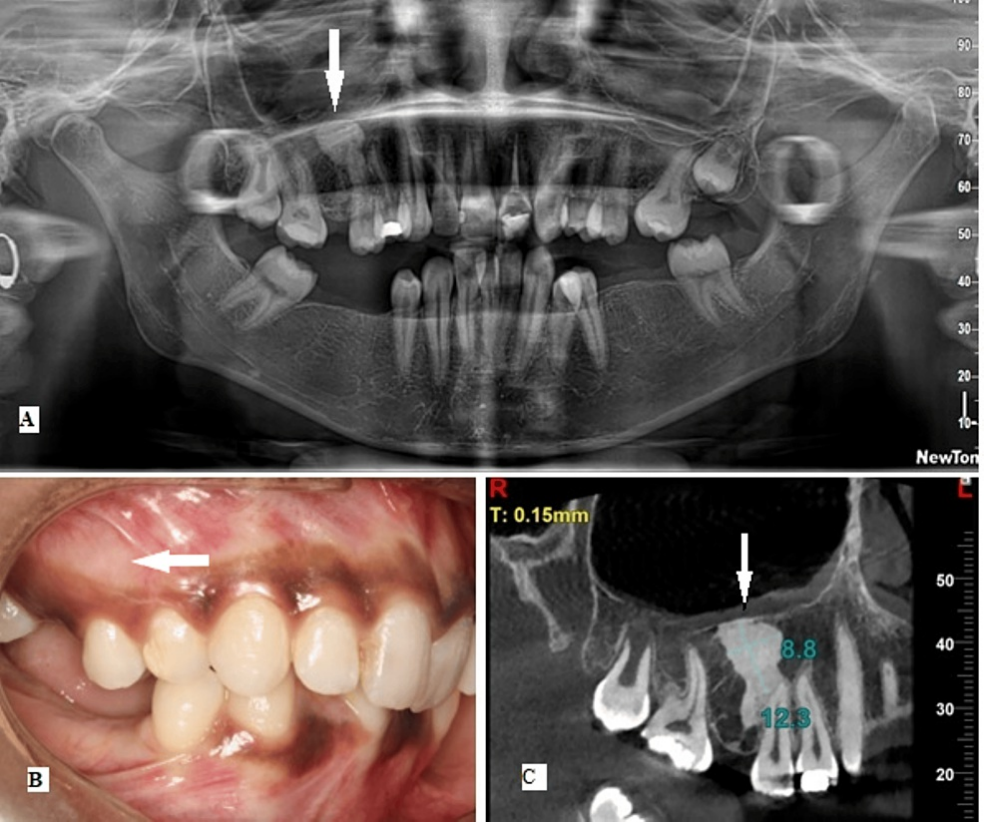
A 19-year-old female presented with multiple missing teeth and malocclusion (A) OPG reveals a solitary round radiopaque mass (arrow), approximately 1 cm in diameter with well-defined margins in the right maxillary second premolar periapical region. (B) The right buccal intra-oral view shows no clinical manifestations (arrow). (C) CBCT image reveals a well-defined irregularly shaped homogeneous radiopacity seen with respect to the periapical region of 15; the lesion was not attached to the tooth and measured approximately 8 × 16 mm in size, extending supero-inferiorly from the floor of the maxillary sinus till the interdental region of 15 and 16. OPG, orthopantomograph
